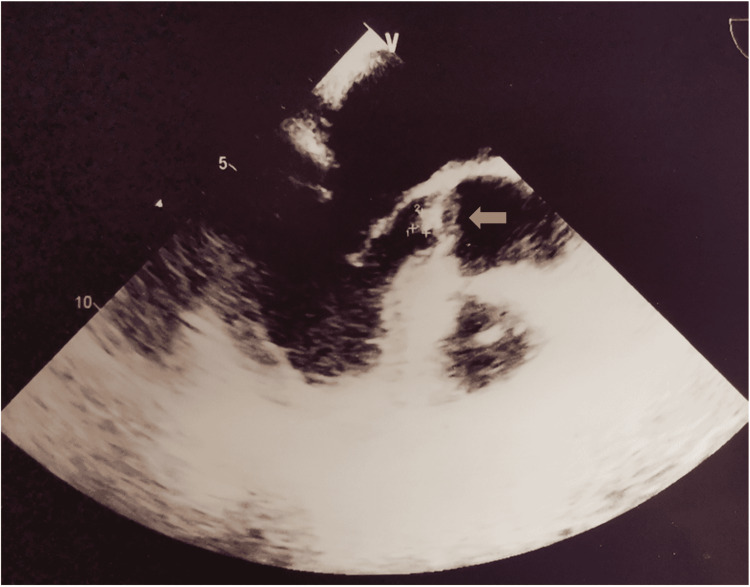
Transesophageal echocardiogram showing a tricuspid valve vegetation measuring 5.3 x 5.6 mm
